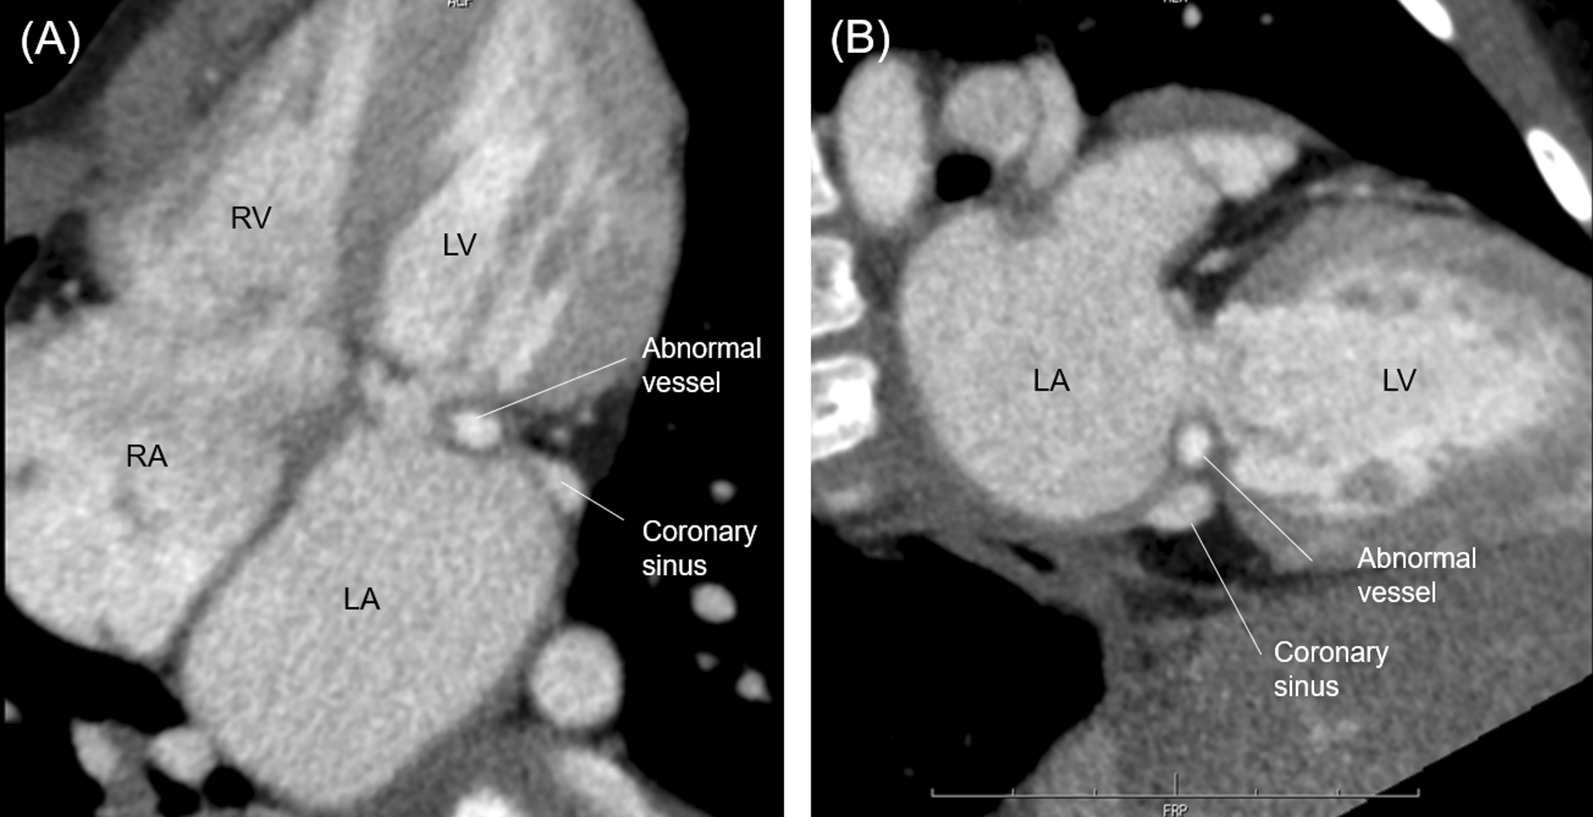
Computed tomography of the patient at 5 years of age revealing left ventricular inflow obstruction caused by an abnormal vessel. a Four-chamber image. b Two-chamber image
